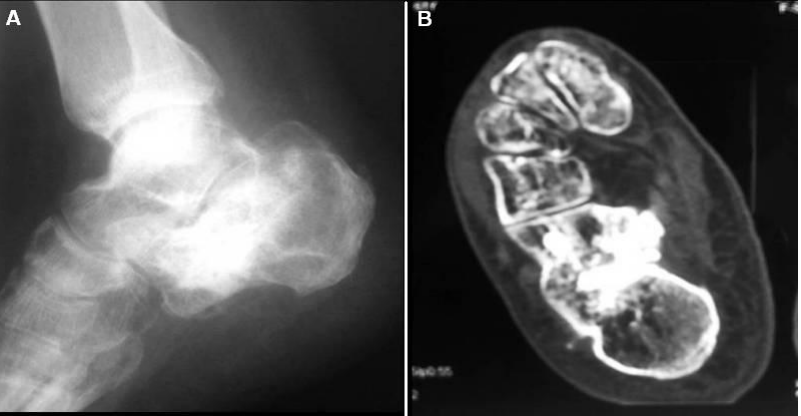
Two years postoperative (a) lateral radiograph and (b) CT scan demonstrating no loss of reduction.
